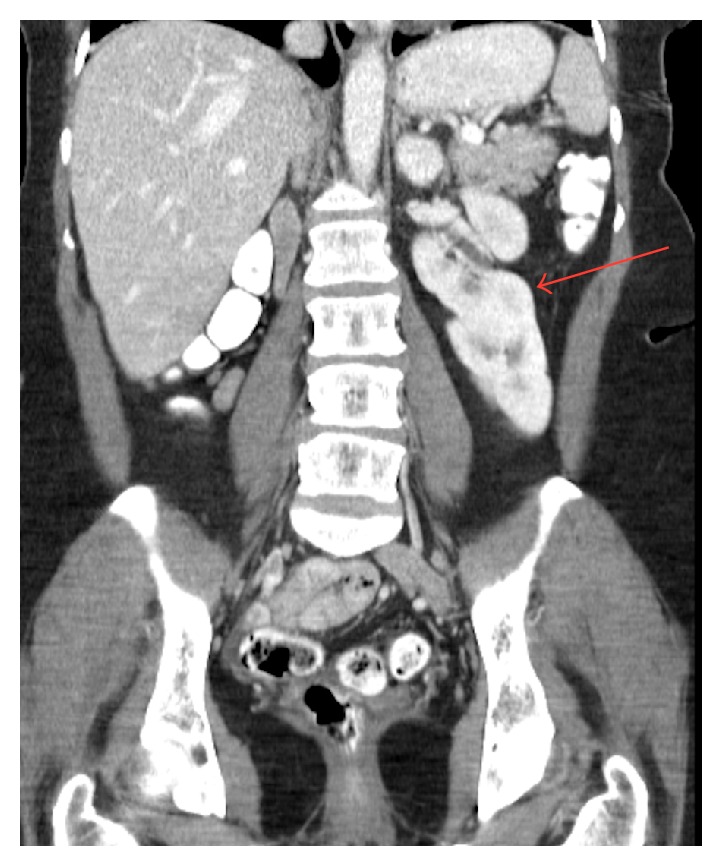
A coronal contrast-enhanced CT image shows an ectopic right kidney fused to the lower pole of the left kidney.
